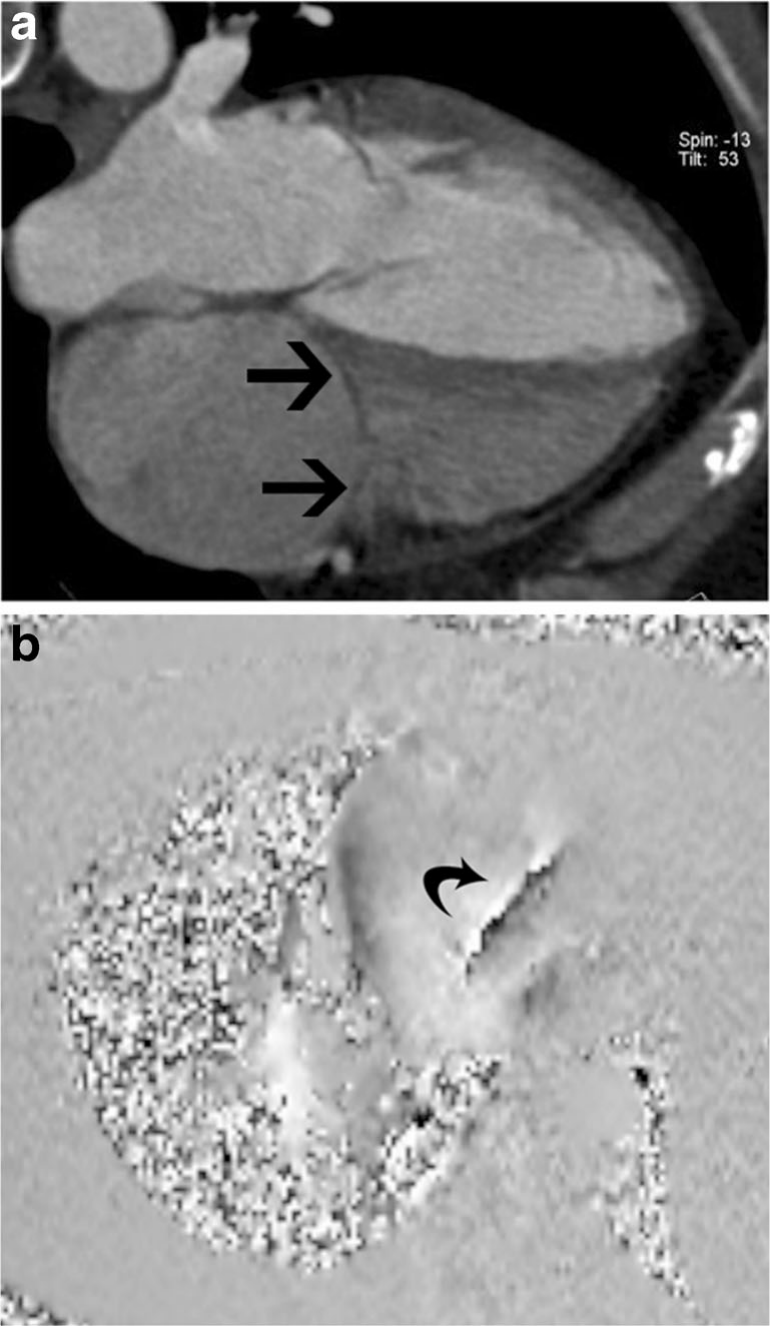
Tricuspid stenosis in CT and MRI. (a) Four-chamber reconstructed CT image shows thickening of the tricuspid leaflets (*arrows*) in a patient with carcinoid and tricuspid stenosis. (b) Four-chamber phase contrast velocity-encoded image shows a high-velocity jet extending across the tricuspid valve, resulting in aliasing (*arrow*)
